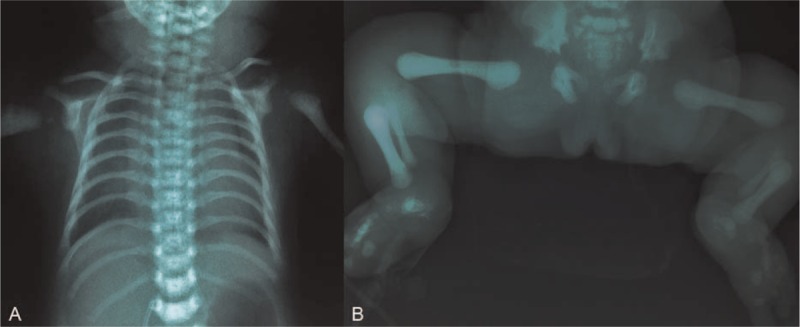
Frontal (A) chest radiograph of case 7 shows narrow thorax with slightly short ribs. The cardiomegaly is due to cardiac defect including atrial septal defect, ventricular septal defect, and patent ductus arteriosus. The vertebral bodies are unremarkable. Radiograph of lower limbs and pelvis (B) shows vertically shortened ilia, and their inferior margins, which are horizontal with a downward-pointing hook at the medial and lateral aspects of the acetabulae (trident or triradiate acetabulum). Femora and tibiae are short and broad, and their lower ends and proximal ends of tibiae are clubbed. Note that there is progressive distal shortening of limbs leading to mesomelia and acromelia. The appearance of the chest and pelvis closely resembles that of asphyxiating thoracic dystrophy.
